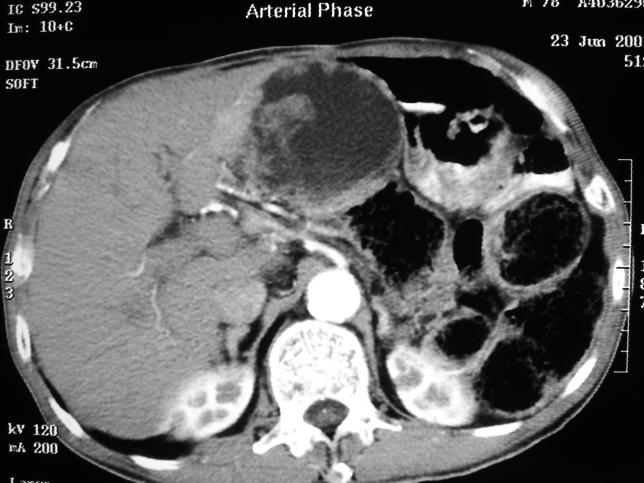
Enhanced Computed Tomography Scan of Abdomen Enhanced computed tomography scan of abdomen showing heterogenous liver metastases with irregular rim enhancement as well as hypodense lesions in the portal region suggestive of metastatic lymphadenopathy.
